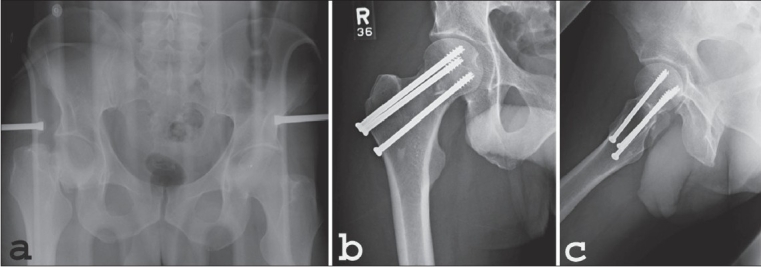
A: AP radiograph of pelvis (a) in a 22 y/o male shows displaced right femoral neck fracture following a motor vehicle accident. (b) Postoperative AP radiograph (c) Lateral hip radiographs after open reduction and internal fixation with three cannulated cancellous screws
